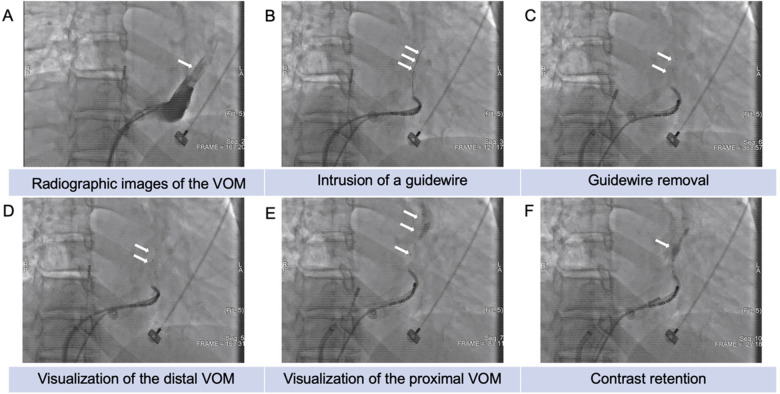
Steps of alcohol ablation of the vein of Marshall (VOM) under x-ray and venography (A) venography visualizing the VOM (white arrow). (B) Intrusion of a guidewire within the VOM (white arrow). (C) Inflation of the OTW balloon in the VOM to 6–8 atm, followed by guidewire removal. (D) Injection of contrast agent through the OTW balloon to visualize the distal VOM (white arrow). (E) Injection of contrast agent through the OTW balloon to visualize the proximal VOM (white arrow). (F) Contrast retention in the VOM region after ethanol injection (white arrow).
